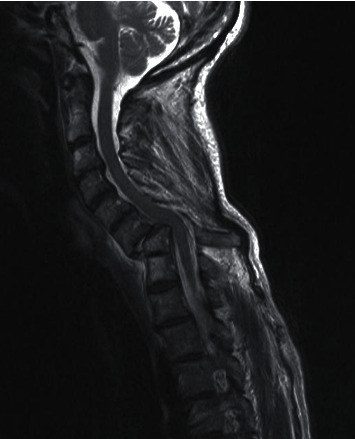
Preoperative MRI scan (sagittal cut).
